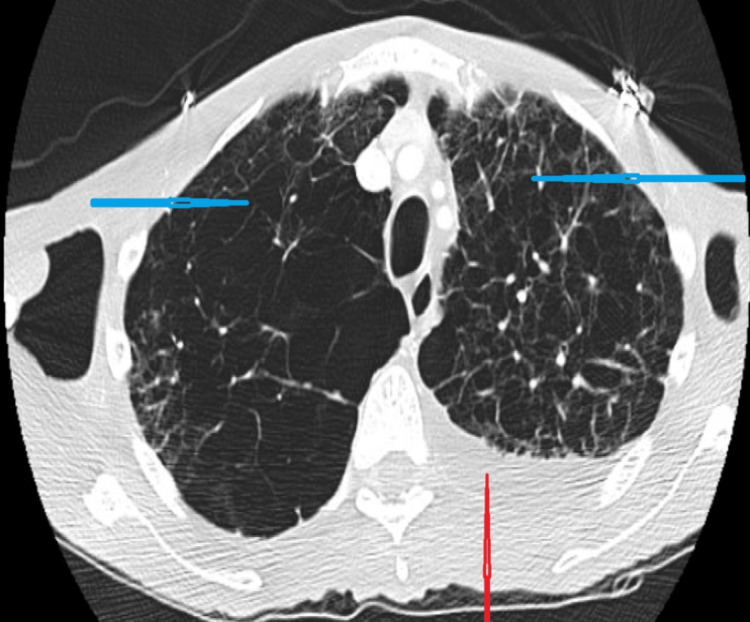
Computed tomography (CT) of the chest in axial section showing severe upper lobe emphysematous changes (blue arrows) and left pleural effusion (red arrow)
